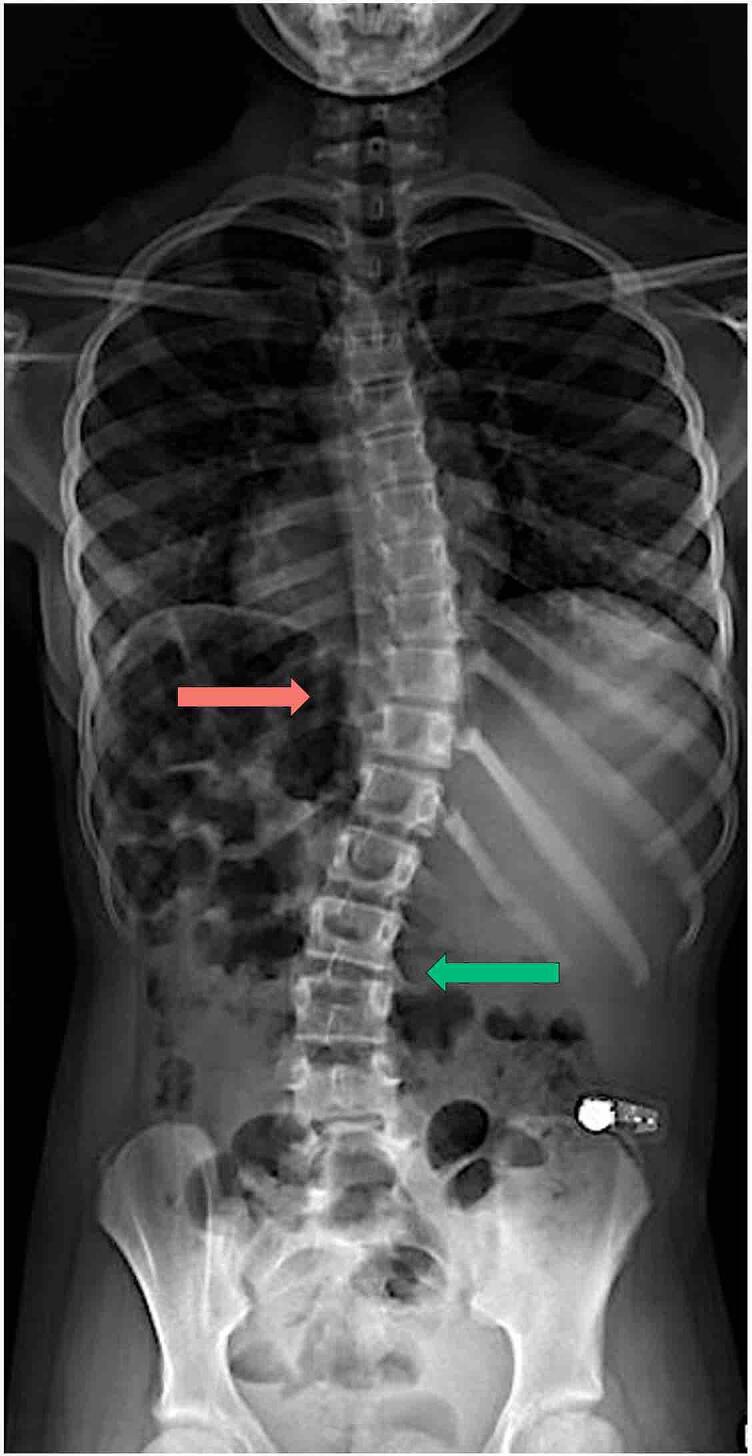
Standing radiograph depicts mild scoliosis (age 11.9y). Findings include dextrocurvature of the thoracic spine (19°) (red) and levocurvature of the thoracolumbar spine (21°) (green). Scoliosis was first incidentally noted from chest radiographs conducted for routine pulmonary care at age 9.2y. For interpretation of the references to color in this figure legend, the reader is referred to the web version of this article.
